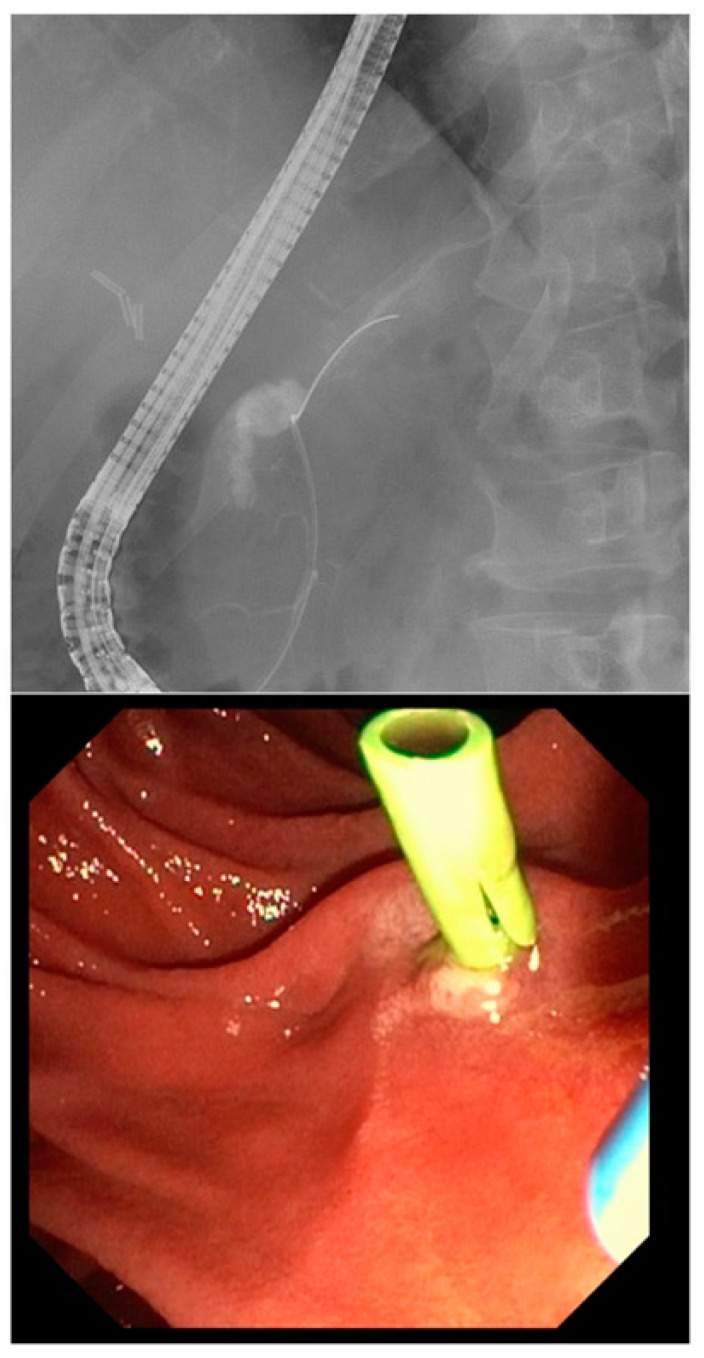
Radiograph of a pancreatic fistula during ERCP and transpapillary stenting.
